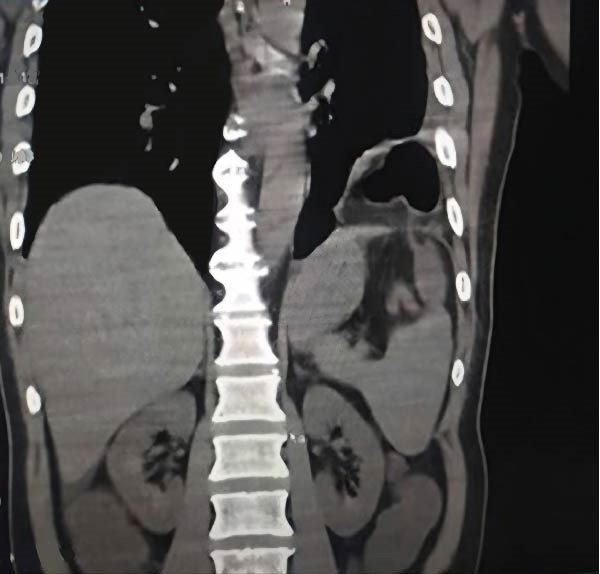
A coronal CT image showing the incarceration of the splenic flexure with omentum from the abdomen within a diaphragmatic hernia.
